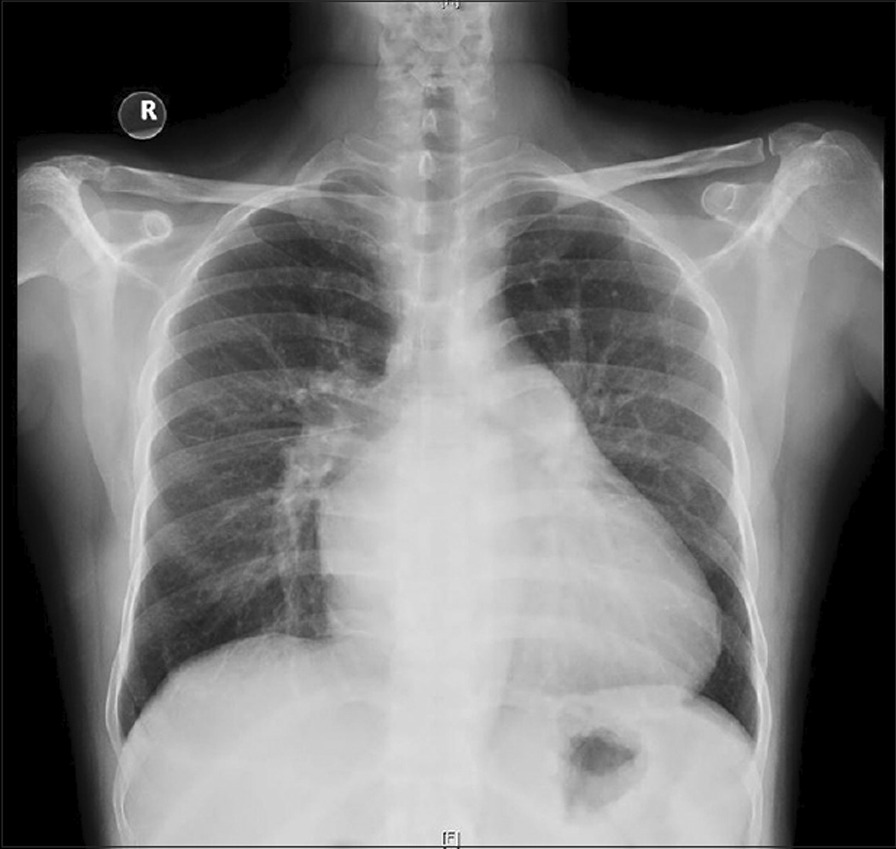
Chest radiograph, showing cardiomegaly and left atrial enlargement
